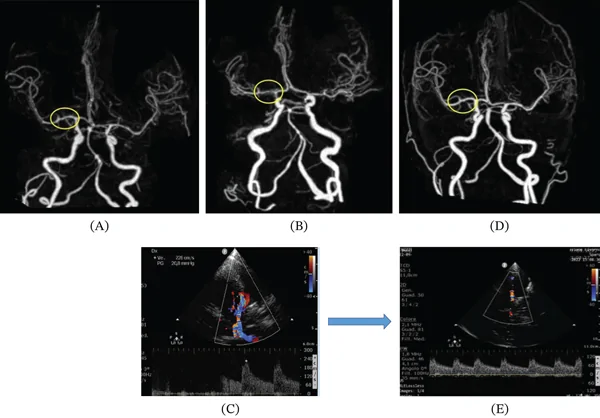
(A–B) CT angiography showing the vessel narrowing. (C) Increase of mean flow velocity in the right middle cerebral artery up to 200 cm/s at TCCD. (D–E) Normalization of flow velocities and vessels diameters after combined oral verapamil and levosimendan.
